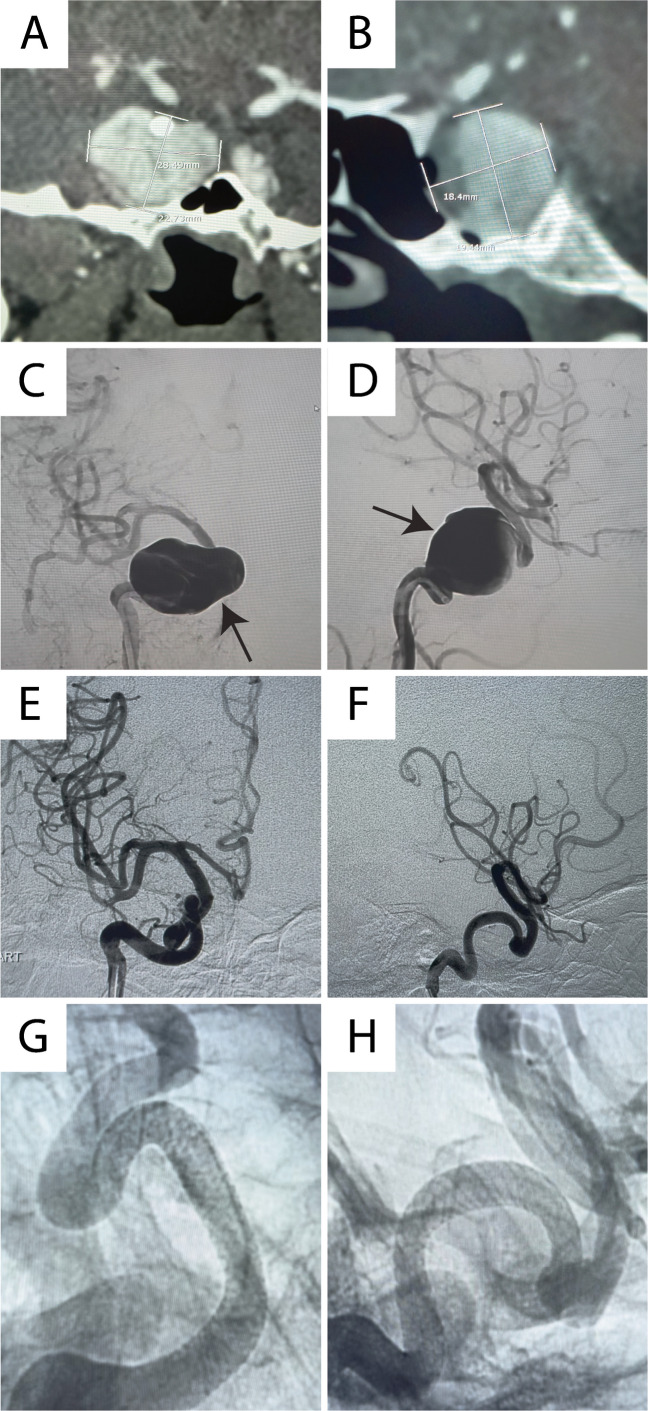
54-year-old Hispanic female with giant right internal carotid artery (ICA) cavernous aneurysm presenting with interval aneurysm growth and development of right sixth nerve palsy. A, B Coronal and sagittal computed tomography angiography (CTA) reconstruction views demonstrating a 22.8 × 26.8 mm (AP projection) and 24.8 × 21.3 mm (lateral projection) partially thrombosed giant right ICA cavernous aneurysm remodeling the sella turcica with expansion and bony erosion. C, D Pretreatment anteroposterior (AP) and lateral angiographic views of right ICA demonstrating the giant right ICA aneurysm (black arrow). E, F Six-month follow-up post-treatment AP and lateral angiographic views of right ICA demonstrating complete aneurysm obliteration with remodeling of the right ICA. Notably, there is improved filling of the intracranial circulation due to resolution of flow steal into the giant aneurysm. G, H Magnified oblique unsubtracted AP and lateral views of right ICA demonstrating the braid of the single Surpass Elite device (4.0 × 30 mm) and smooth remodeling of the cavernous right ICA with no residual aneurysm filling
